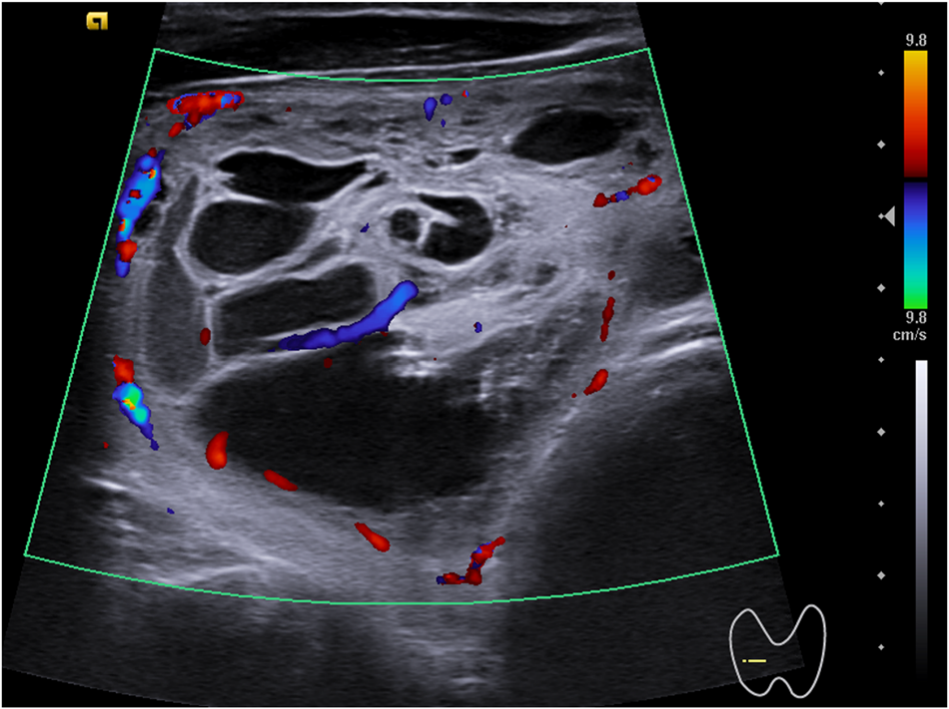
Ultrasound presentation of one case of MTC. The nodule is placed in the right thyroid lobe and presents as mixed with isoechoic and anechoic parts, mildly vascularized, with largest diameter of 44 mm.
